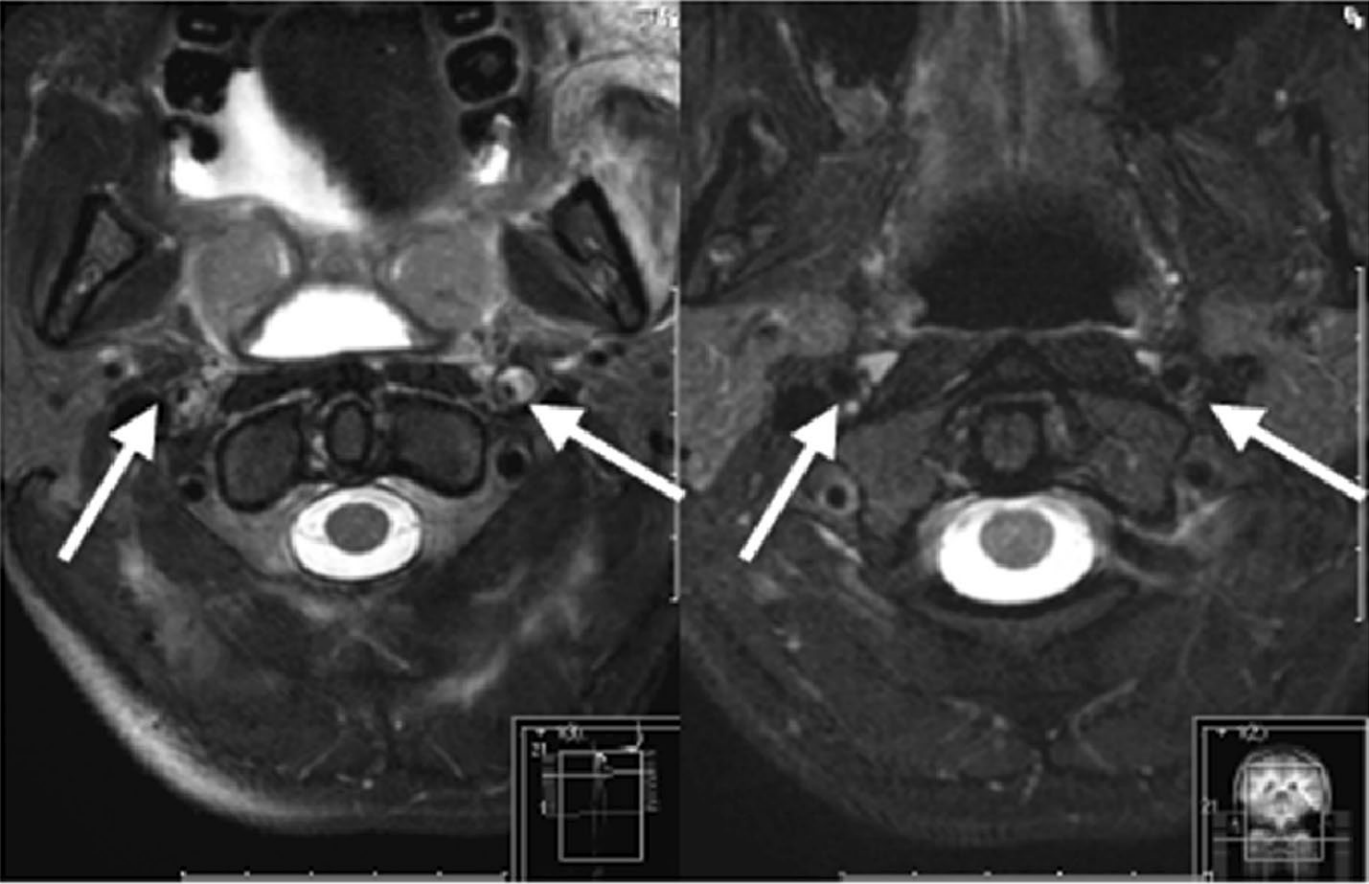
Axial T-2 sequences of a brain MRI Angio scan of the 28-year-old patient. *Left* posttraumatic intracranial dissection with intramural hematomas of both internal carotid arteries 3 days after trauma (indicated with *arrows*). *Right* 6 months follow-up MRI scan with good perfusion of both internal carotid arteries.
